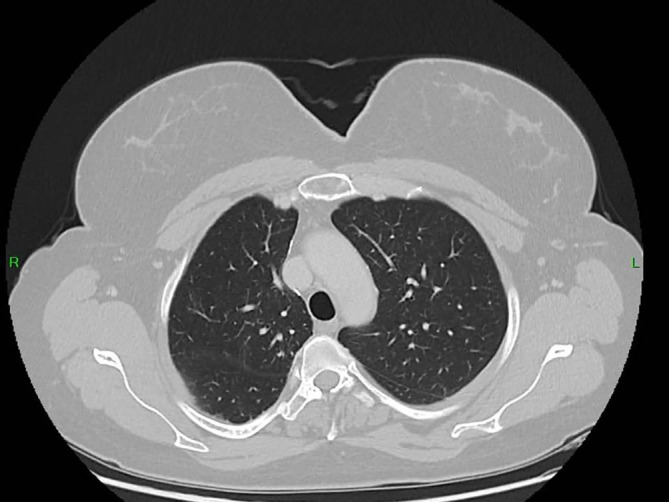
Follow‐up chest CT image obtained 18 months after video‐assisted thoracoscopic surgery (VATS) with right upper lobectomy and lymphadenectomy, and before initiation of pembrolizumab immunotherapy, showing no radiological evidence of recurrent thoracic disease.
